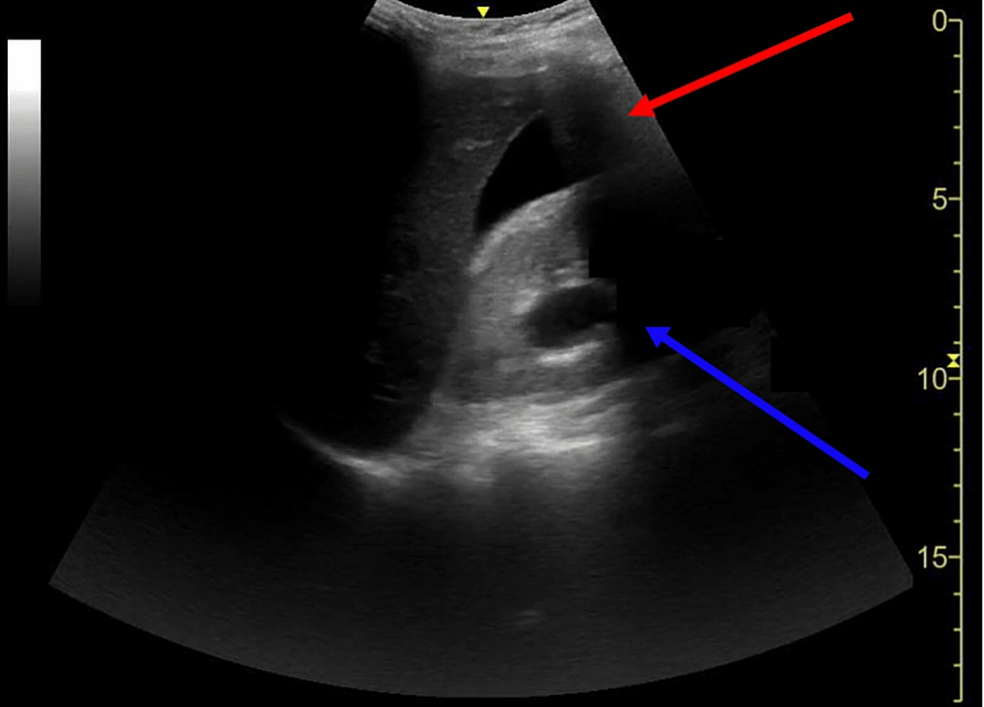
Sonographic image of the right upper quadrant Bedside POCUS image utilizing a low-frequency curvilinear probe at the right upper quadrant showing intraperitoneal free fluid in Morrison’s pouch in the setting of a newly diagnosed rectal mass lesion (red arrow). There is evidence of hydronephrosis in the right kidney (blue arrow).
